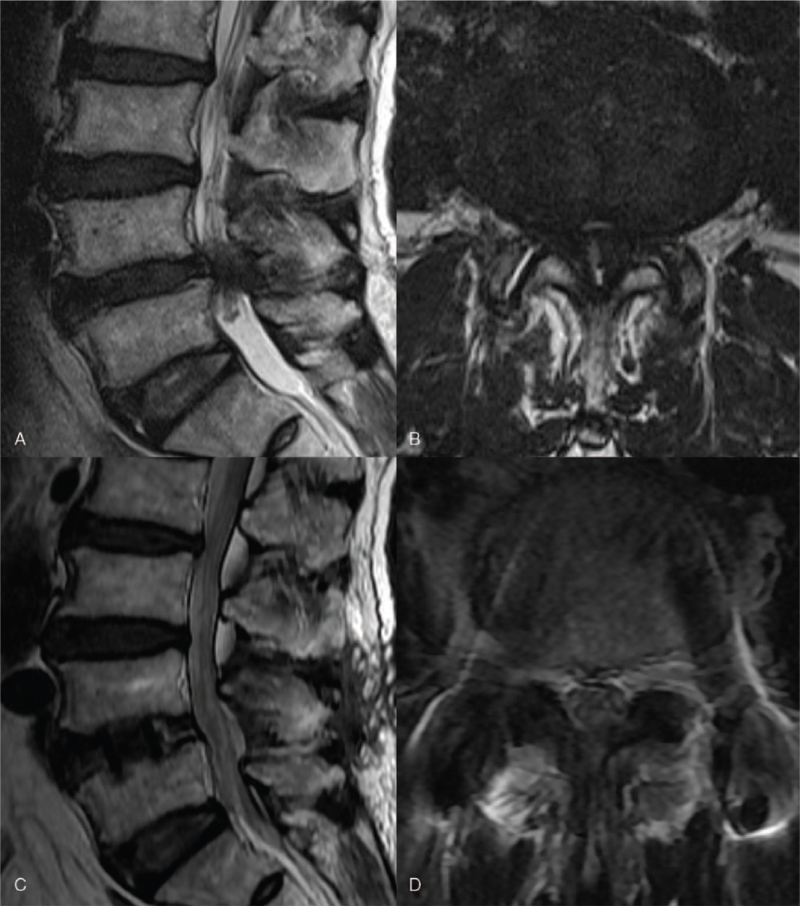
Comparison of the MR images before and after the first surgery (oblique lumbar interbody fusion, followed by bilateral L4 laminotomy and L4-5 percutaneous posterior screw fixation). In January 2018, the patient complained of numbness and pain in the soles of both feet. There was no muscle weakness. (A) Nerve root redundancy is observed in the sagittal view, and (B) severe L4-5 central stenosis is observed in the axial view. In May 2018, the patient presented to the hospital with persistent pain in both ankles and decreased muscle strength in the right ankle. (C) Nerve root redundancy is resolved (sagittal view). (D) Central stenosis is also resolved (axial view).
